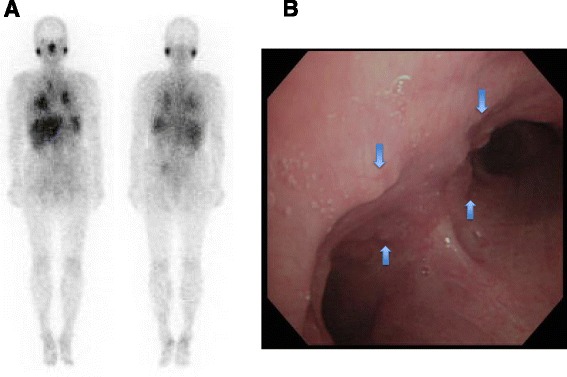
Gallium-67 scintigraphy and bronchoscopic examination. a Gallium-67 scintigraphy exhibits abnormal uptake in the right subclavicular area, mediastinum, bilateral hilum, bilateral parotid glands and spleen but no abnormal uptake in the heart. b A bronchoscopic examination reveals multiple small nodules on the surface of the bilateral bronchi (arrows). A carina is located at the center
